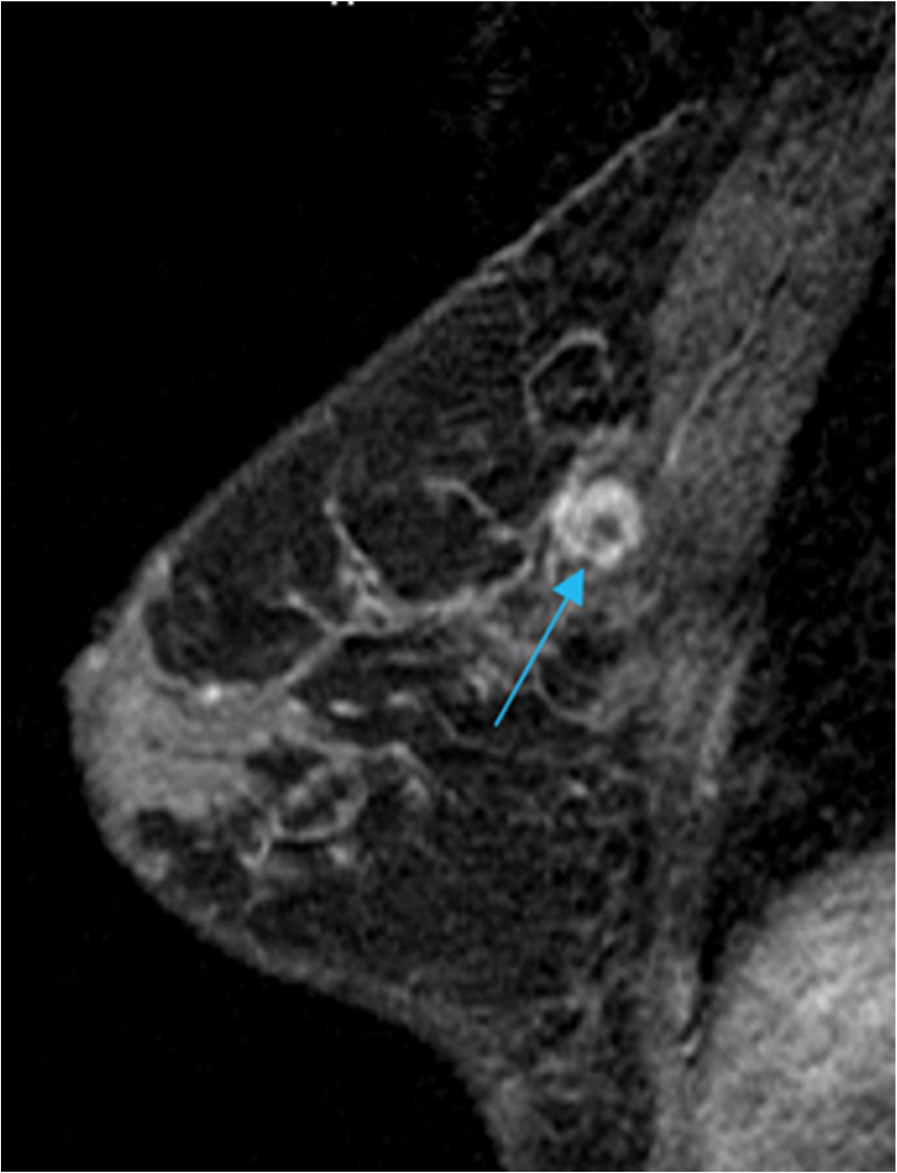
Sagittal fat-suppressed T1-weighted first post-contrast image of a breast cancer (arrow) where computer-extracted image phenotype (CEIP) sphericity is high and the radiologist assessed it as round/oval for shape. CEIP and human-extracted image phenotype are concordant
